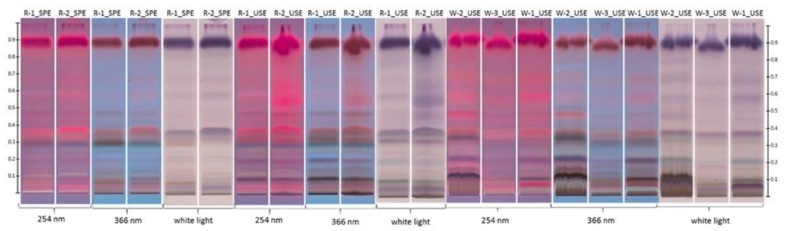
HPTLC fingerprint of rape and willow honeys after derivatisation (UAE—ultrasound assisted extraction, SPE—solid phase extraction visualised under white light, 254 nm and 366 nm; R—rape honeys; W—willow honeys.
